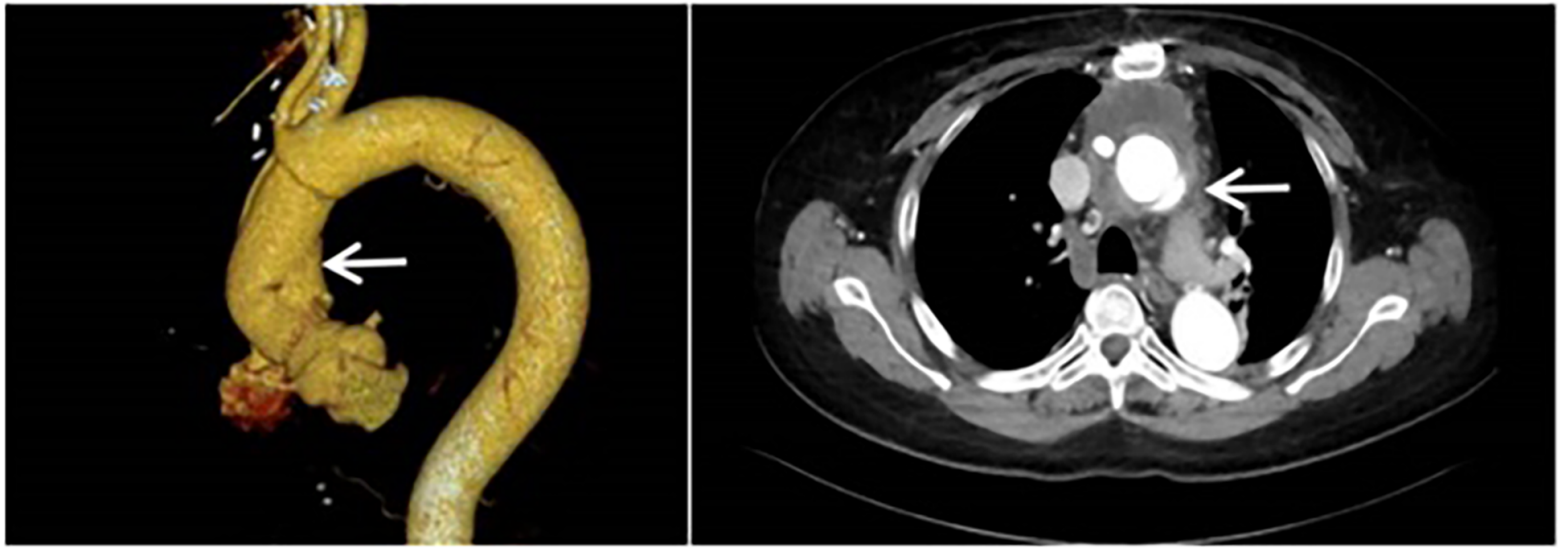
Computed tomography angiography of the aorta was performed when the patient was admitted to the hospital for the first time. (It was indicated that there was type A aortic dissection; the formation of true and false lumens could be seen distal to the origin of the right coronary artery in the ascending aorta).
